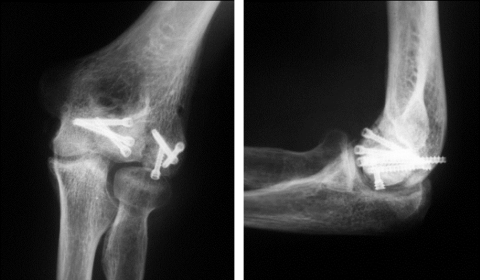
Case 6. Anteroposterior and lateral radiographs taken 6 months after surgery showing minimal bone resorption of the capitellum, moderate lateral compartment osteoarthritis, and partial extrusion of a Herbert screw.
